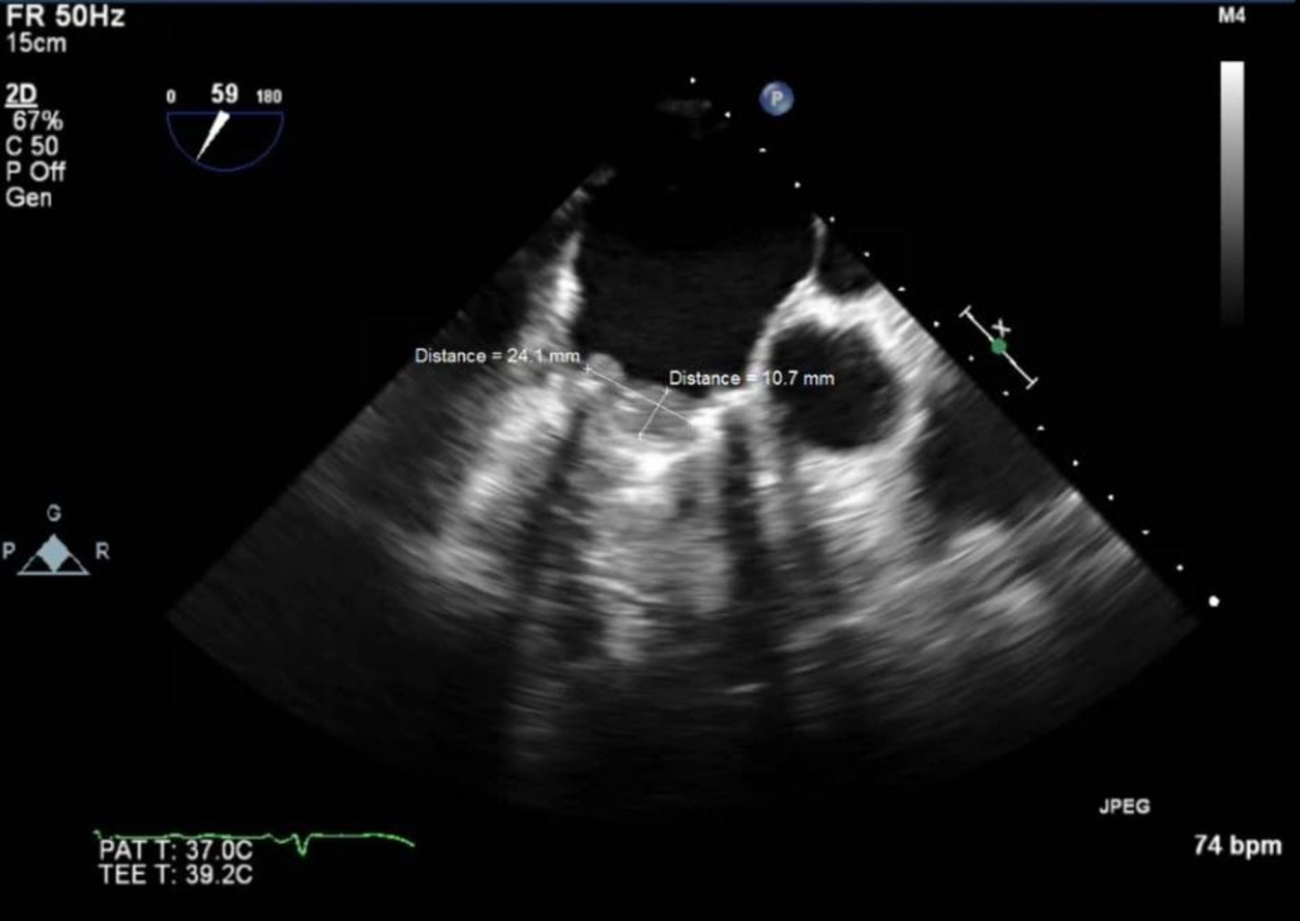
Transesophageal echocardiogram view of the tricuspid valve revealing a thrombus measuring 2.41 cm x 1.07 cm along the atrial aspect of the mechanical tricuspid valve
